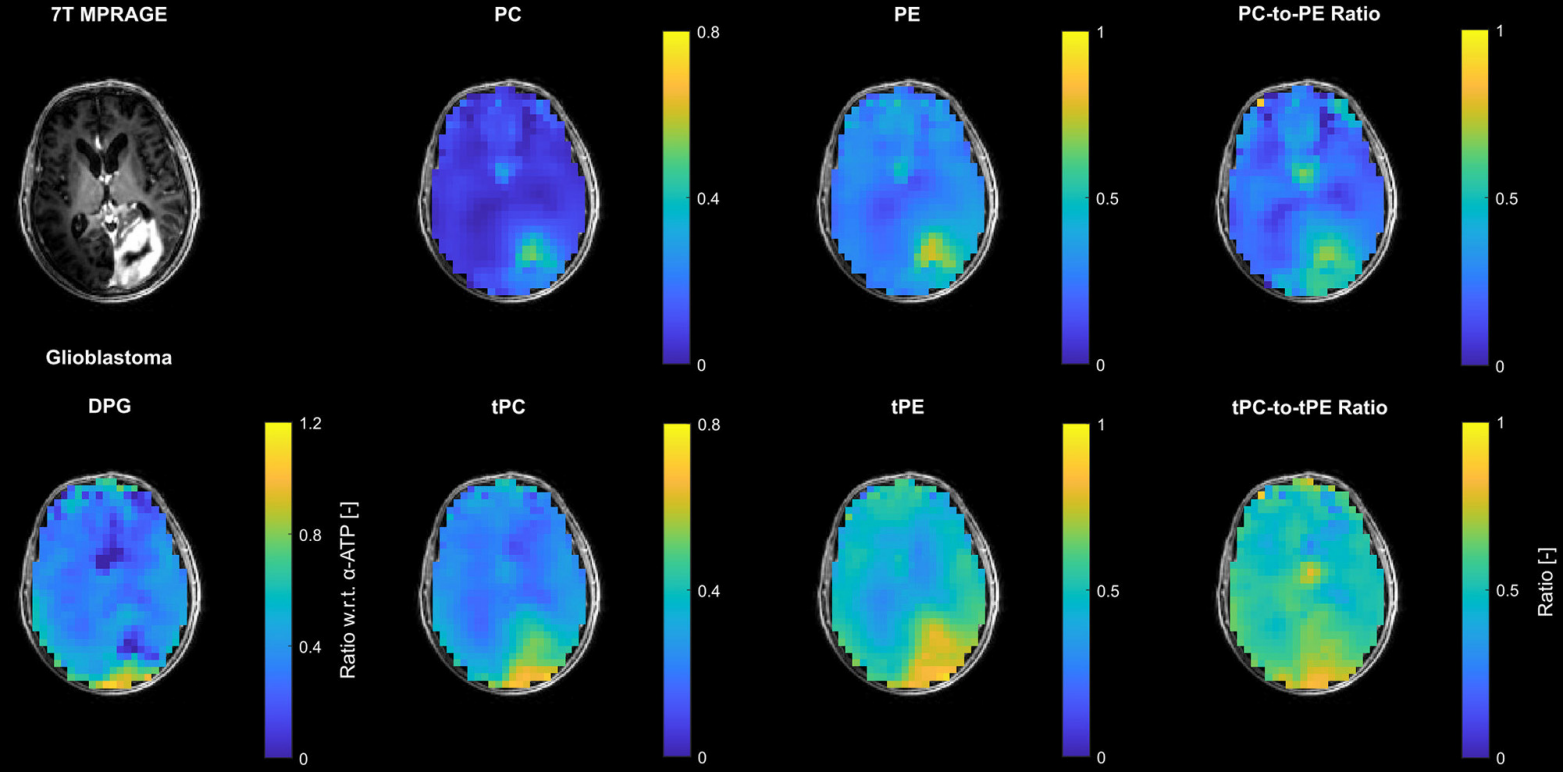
Representative transversal slices of 3D metabolite maps quantified in the PME region from the patient with high-grade glioma (glioblastoma; cf. Figure 1C), shown as ratios with respect to the α-ATP intensity. Note that the scales for the actually modeled (PC, PE) and the corresponding surrogate intensities (tPC, tPE) are the same. For visual guidance, the metabolite maps are masked to show only brain parenchyma as displayed on the 7T non-enhanced T1-weighted MPRAGE images.
